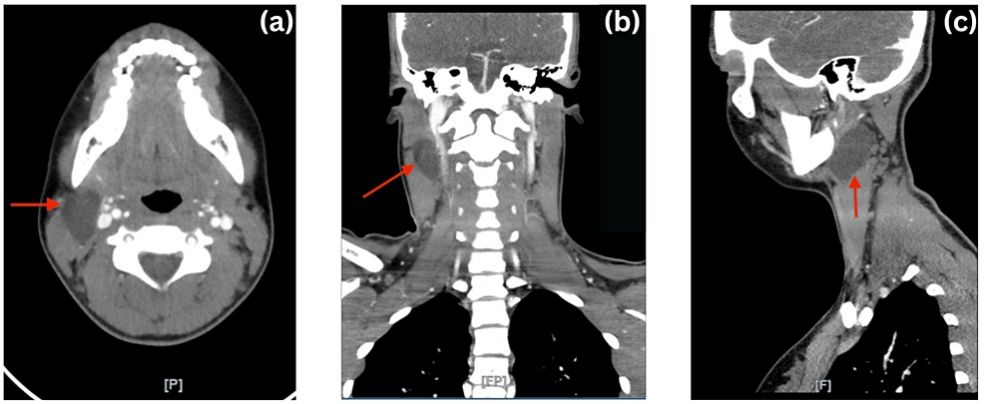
CT with contrast showing the lesion (red arrow) in the axial, coronal, and sagittal sections. The red arrow points towards the hypodense lesion, which measures 31x22x20 mm. The lesion had a uniform density and was observed in the right upper third of the neck, deep in the SCM muscle at the lower end of the parotid gland. It abutted the carotid sheath without infiltration and had neither calcifications nor septations.
